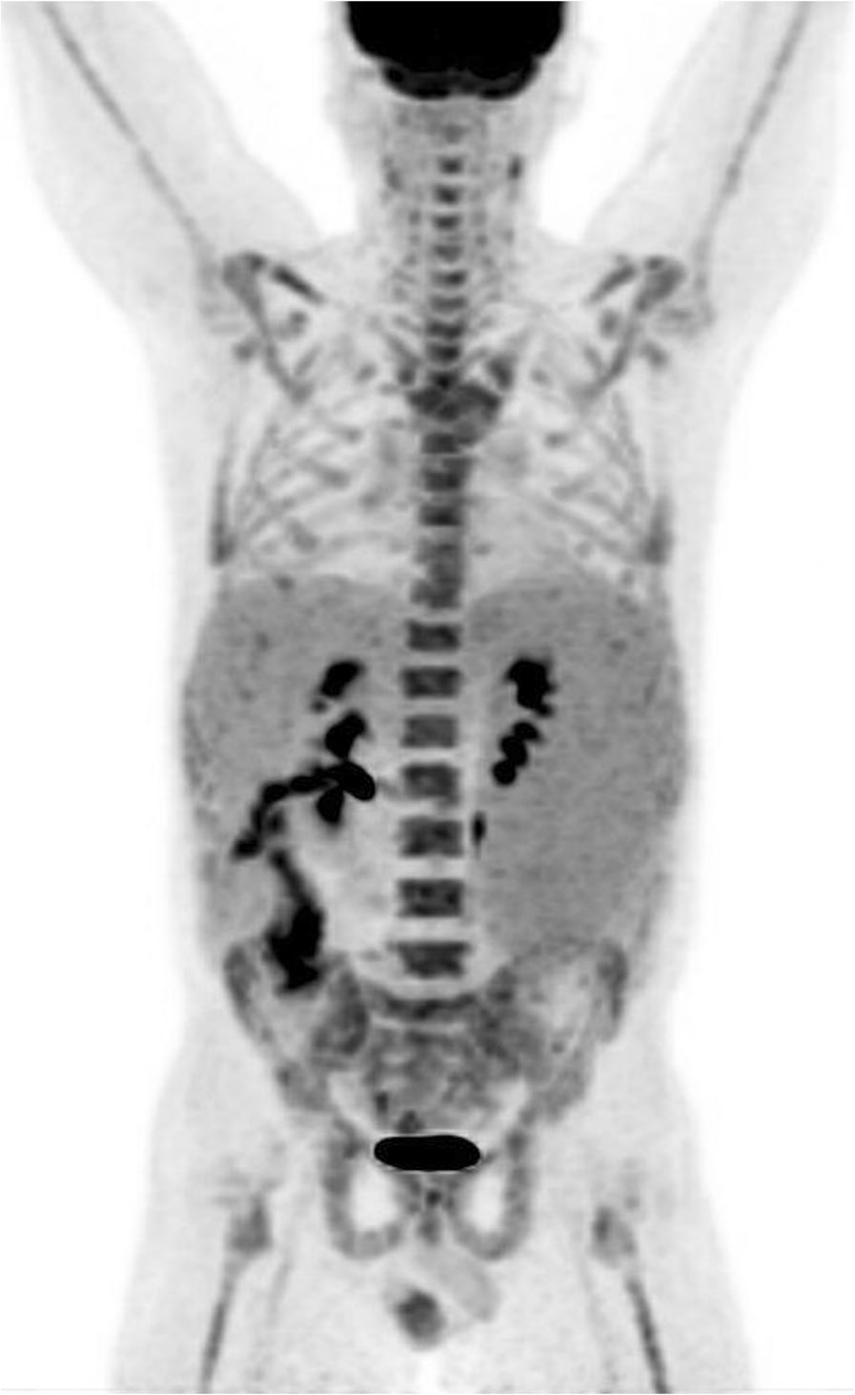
^18^F-FDG PET/CT MIP image of a patient with myelofibrosis demonstrates diffuse FDG uptake in the bones. Note the presence of hepatosplenomegaly in the patient
